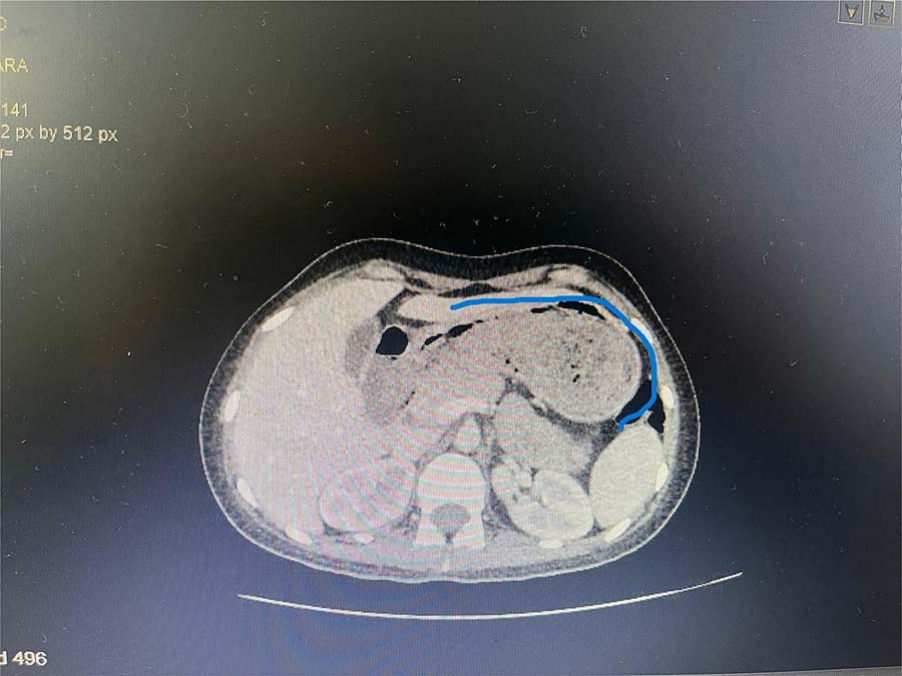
Axial CT image showing the foreign body completely occupying the gastric lumen.
